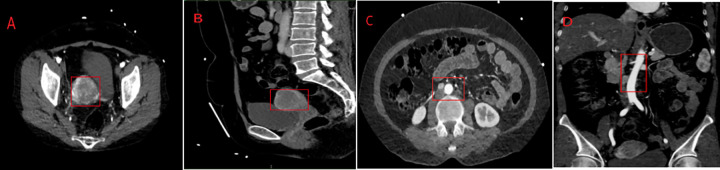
Preoperative imaging. Pelvis CT showed (A, B) thickening and enhancement of the endometrium. Abdominal enhanced CT scan (C, D) showed increased retroperitoneal and bilateral perivascular lymph nodes with partial enlargement. Red box: Indicates lesion and increased lymph nodes.
